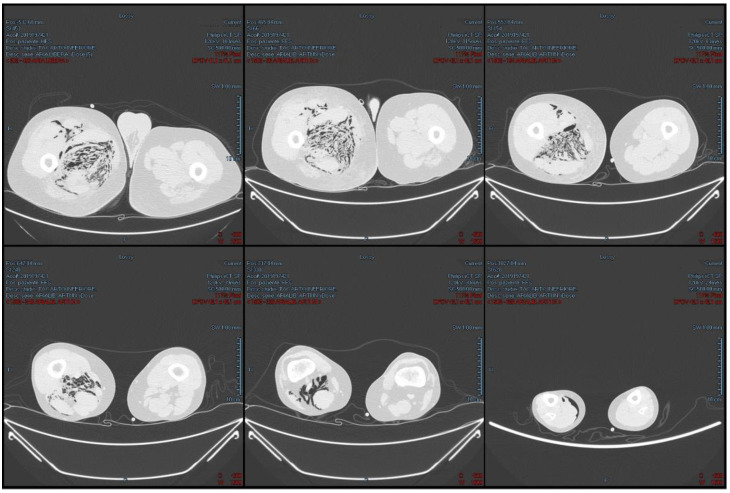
Pre-operative axial CT-scan of the lower limbs and lung window.
